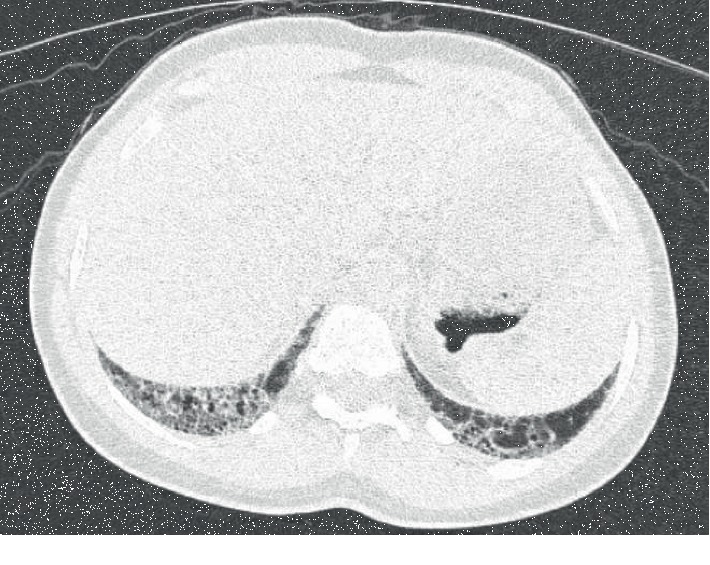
HRCT chest, prone image, honeycombing, and reticulation peripheral lung bases.
